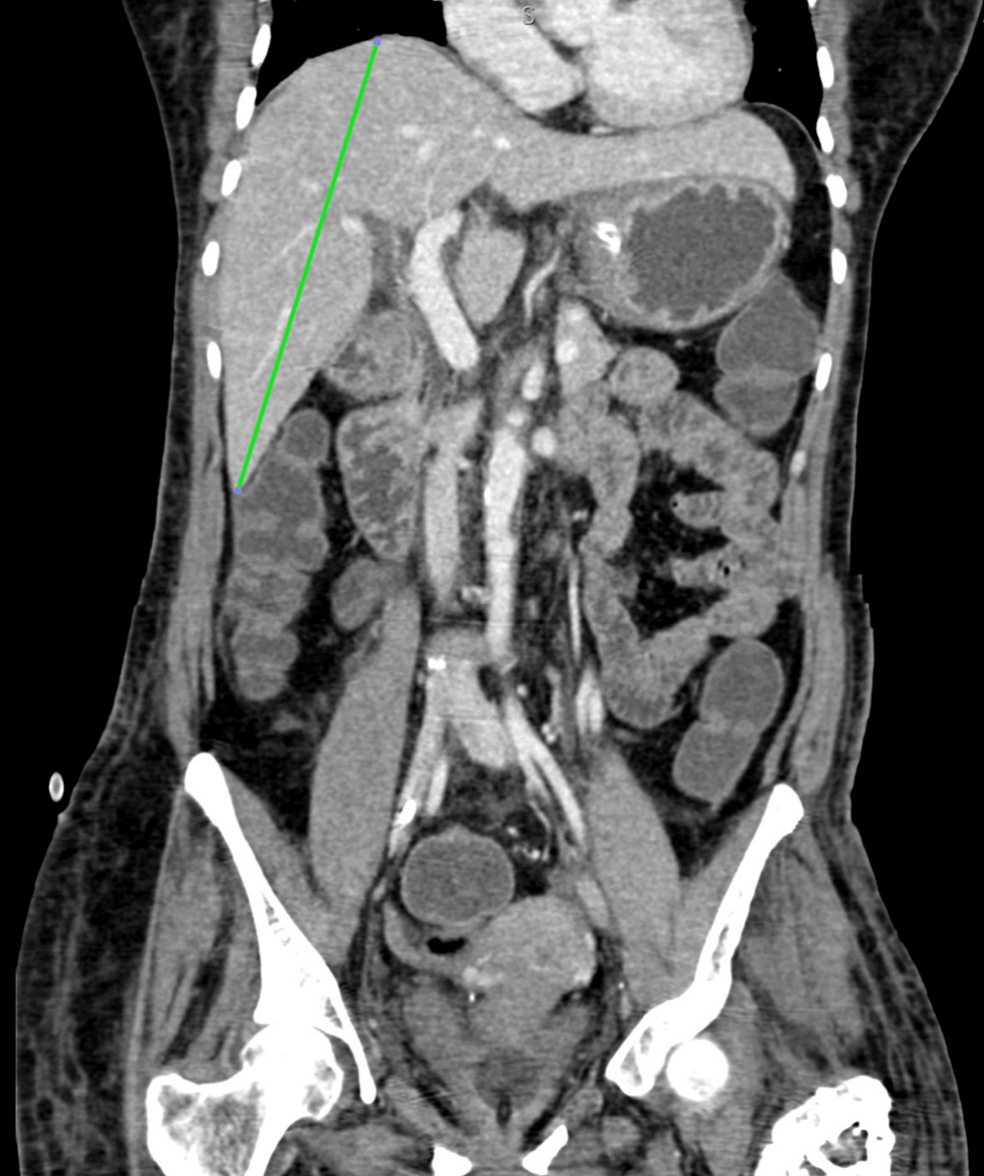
Contrast-enhanced computed tomography (CECT) of the abdomen (sagittal view) The green line shows hepatomegaly
